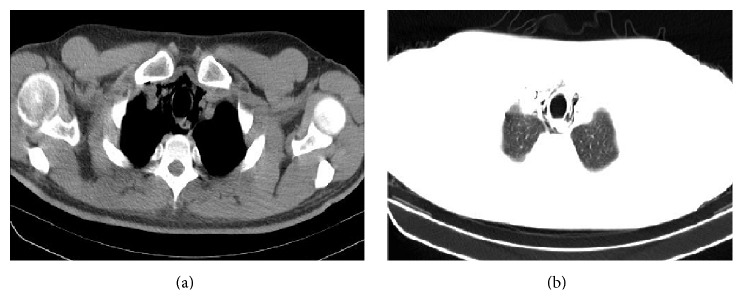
The view of mediastinal emphysema in thorax CT.
